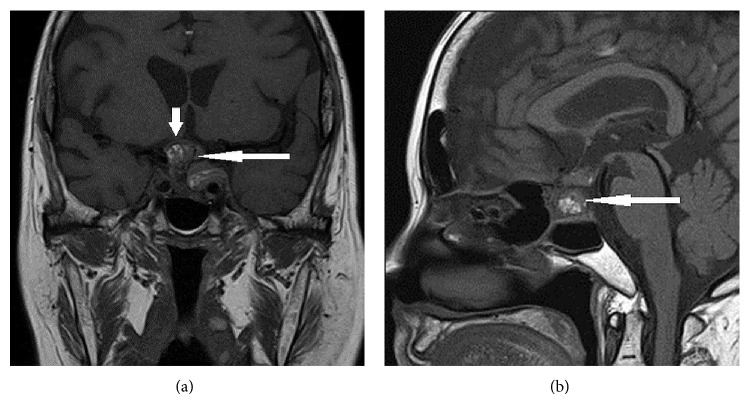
(a) MRI pituitary (T1-weighted, contrast, and coronal) showing pituitary mass (long arrow) with mass effect on the optic chiasm (short arrow). (b) MRI pituitary (T1-weighted, contrast, and sagittal) showing presence of increased signal intensity in the pituitary mass, representing presence of blood products (long arrow).
